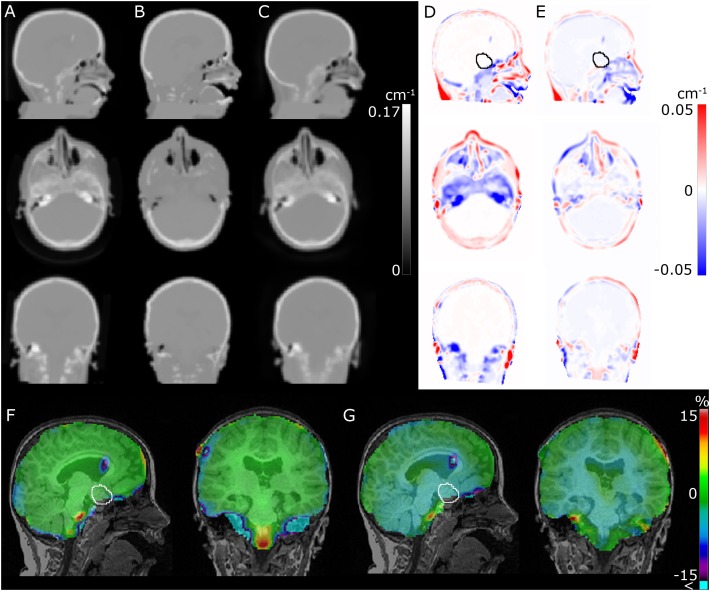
Comparison of CT (A), RESOLUTE (B), and DeepUTE (C) attenuation maps in the sagittal, axial and coronal orientation, respectively. (D,E) shows (B,C) subtracted (A), respectively, and (F,G) shows the resulting relative difference in the PET images between RESOLUTE and DeepUTE relative to CT-AC, respectively. The improved accuracy in the nasal cavities, the skull base and the mastoid processes, leads to a clear reduction of the errors in the surrounding regions, e.g., in the medulla. It also appears that, for this patient, a small underestimation of the densities within the brain in DeepUTE leads to a small underestimation globally within the brain. The tumor delineation is show on the sagittal view in (D–G).
